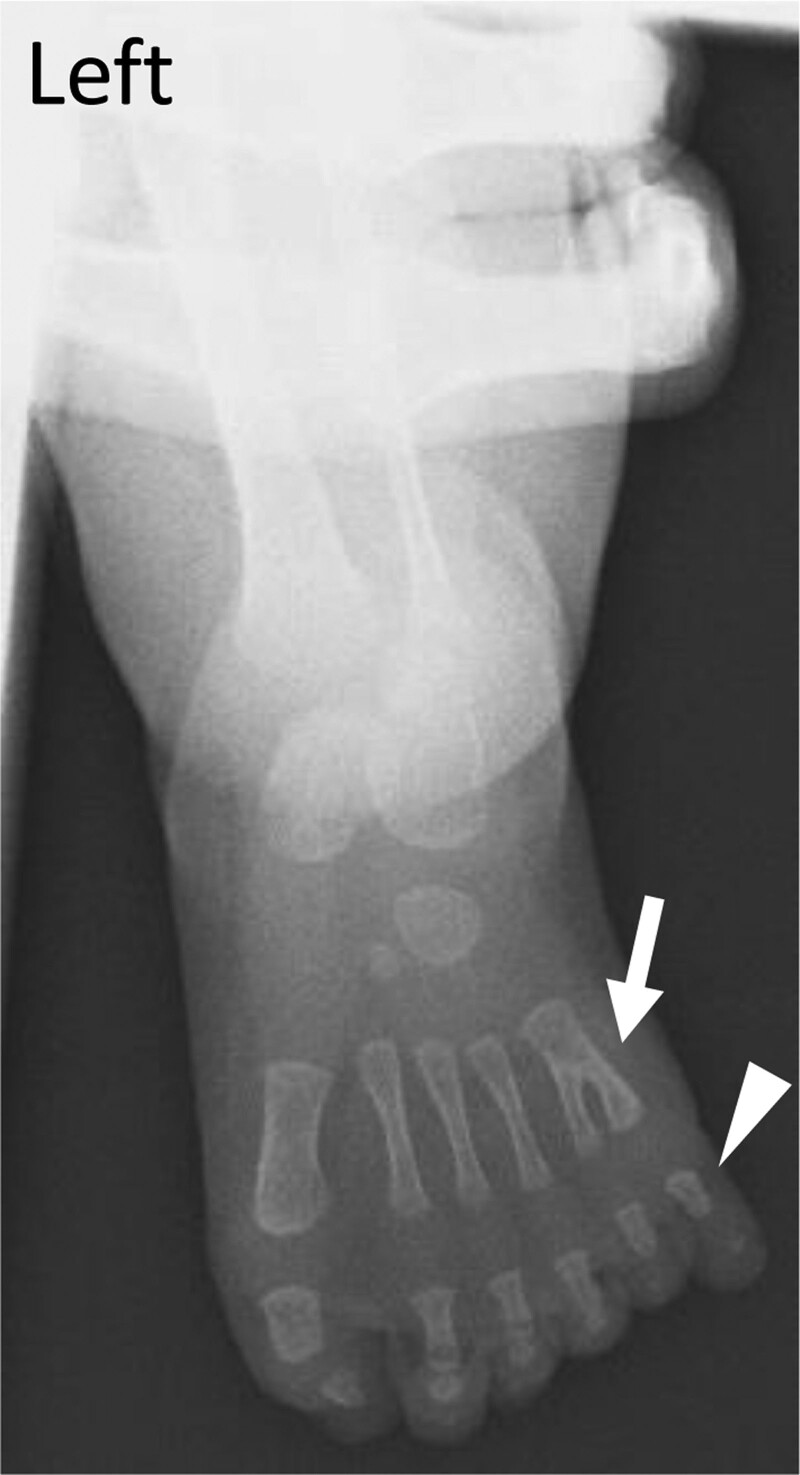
X-ray image of the left foot at birth showing polydactyly (arrowhead) and synpolydactyly (arrow) on his left foot.
